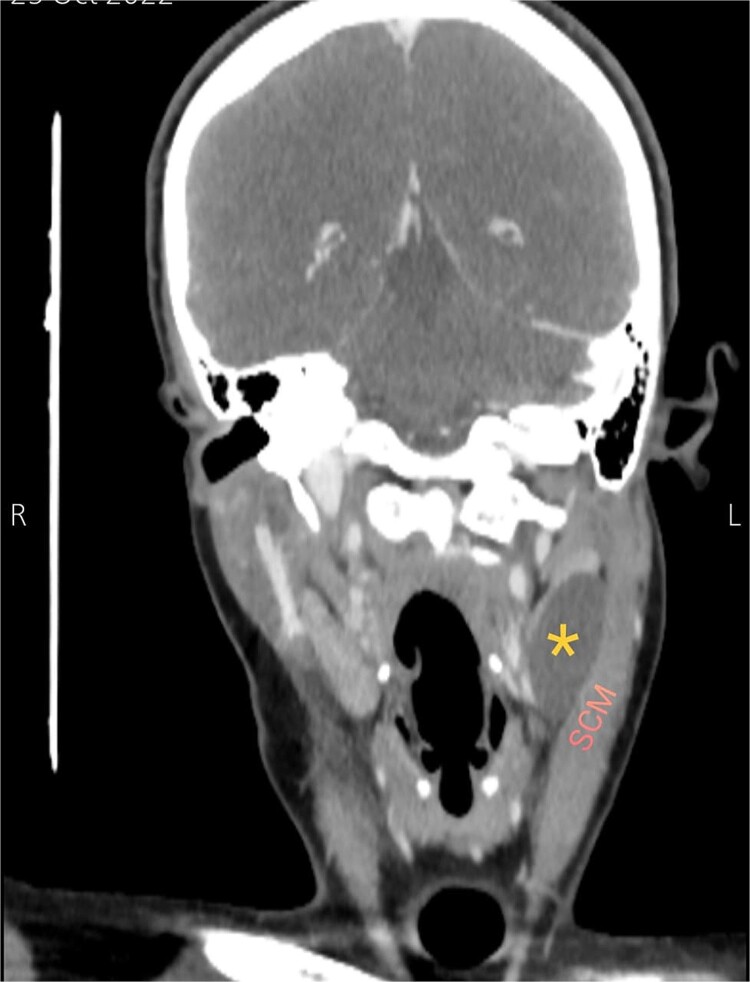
Coronal CT neck scan showing the branchial cleft cyst lying beneath the SCM. Asterisk: branchial cleft cyst.
